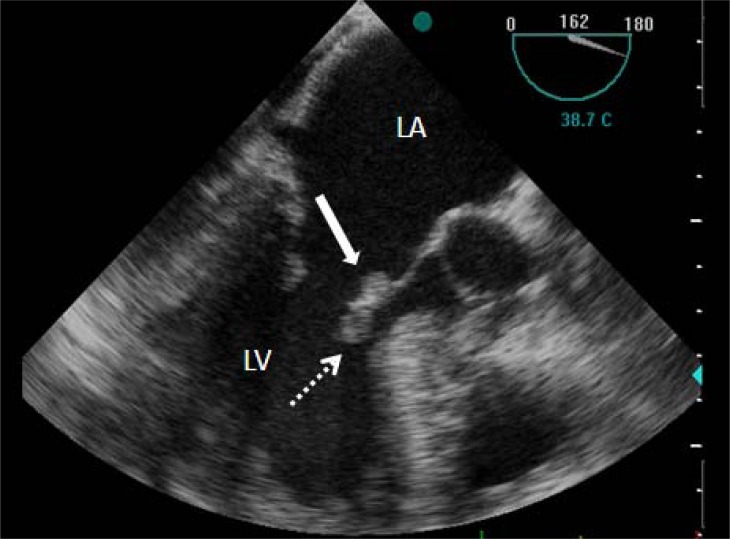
Transesophageal modified long-axis view showing vegetations on both atrial (arrow) and ventricular aspects (dotted arrow) of the mitral anterior leaflet (LA: left atrium, LV: left ventricle).
